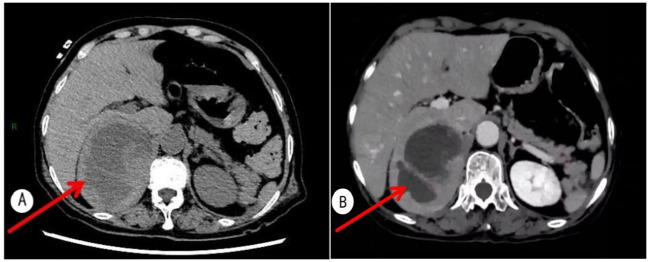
(A) Adrenal CT shows a mass lesion with heterogeneous density in the right adrenal region and minimal exudative changes around the right kidney. (B) Contrast-enhanced adrenal CT reveals a cystic-solid mass in the right adrenal gland (approximately 11.1 x 7.9 cm in size), highly suspicious for pheochromocytoma, with adrenocortical adenoma/carcinoma also requiring consideration.
